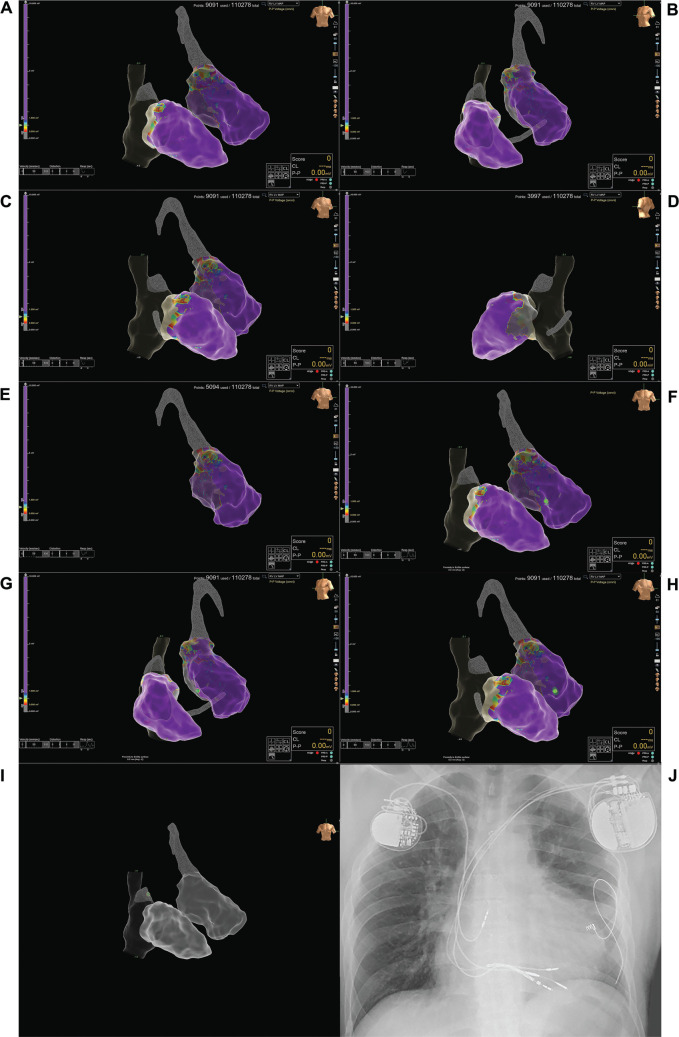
Three-dimensional voltage maps of both ventricles clearly depict the patch area in the basal septal region in the anteroposterior (A), left anterior oblique (B), and right anterior oblique (C) views. The patch area is also shown separately for the morphological left ventricle and morphological right ventricle in the posterolateral (D) and right anterior oblique (E) views, respectively. After implantation deep in the septum, the tip of the pacing electrode in unipolar configuration is seen as a green tag just beneath the scar area in the anteroposterior (F), left anterior oblique (G), and right anterior oblique (H) views. The tip of the atrial pacing electrode in unipolar configuration is observed as a green tag in the right atrial appendage (I). A chest X-ray displays the left-sided implanted cardiac resynchronization device with the atrial, septal, and defibrillator electrodes. The right-sided older generator and pacing electrodes, endocardially on the right side and epicardially on the left side, are visible (J). In the voltage maps, the gray area represents the right atrium, superior vena cava, and inferior vena cava, while meshed gray areas indicate the right atrial appendage and coronary sinus on the right side and the aorta on the left side.
